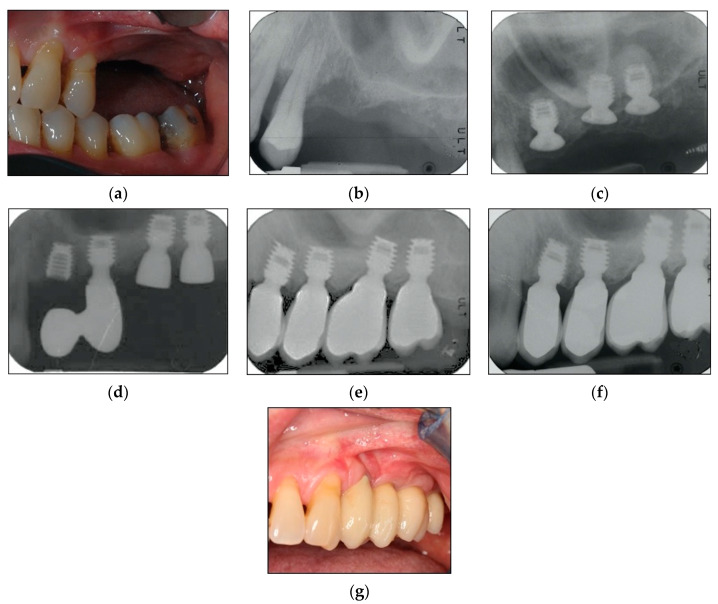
(a–g). Clinical case: Four implants placed in 2.4, 2.5, 2.6, and 2.7 sites. (a) Clinical photograph before implants placement. (b) Pre-operative radiograph before implants placement in sites 2.5, 2.6, and 2.7. (c) Radiograph obtained at implants placement. See augmented sinus floor. (d) Radiograph obtained at loading time. Another implant was placed in site 2.4 (the extracted tooth had grade 3 mobility and was extremely compromised). Site 2.4 was temporarily restored using the implant in 2.5 site to support a cantilever prosthesis. (e) Radiograph obtained at loading time. See definitive restorations for all implants. (f) Radiograph obtained at three-year follow-up. See stable bone levels. (g) Clinical photograph at three-year follow-up. See stable clinical conditions.
